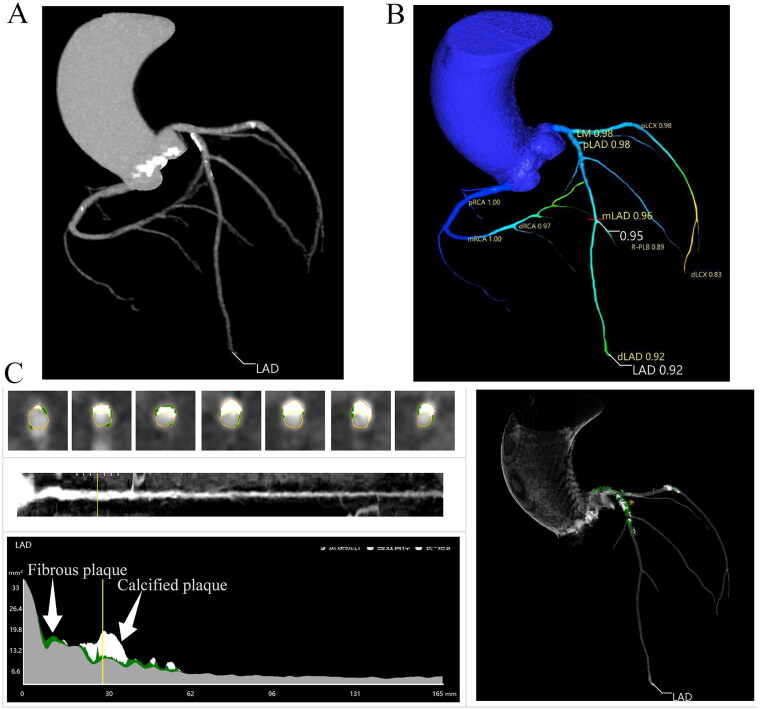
Representative images from oblique reformatted coronary CT angiography in a 77-year-old man with severe aortic stenosis. (A) Coronary CT angiography without obstructive coronary artery disease. (B) The final CT angiography–derived fractional flow reserve (FFR) results in major epicardial arteries. (C) The plaque characteristics analysis in LAD. CT: computerized tomography; LAD: left anterior descending artery; LCX: left circumflex artery; RCA: right coronary artery.
